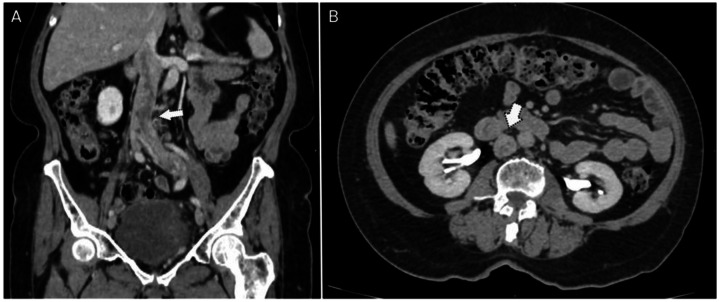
Contrast-enhanced abdominal CT Venous phase (A, B) and delayed phase (C). Filling defect in the left common iliac vein with extension into the inferior vena cava. The left common iliac vein is enlarged compared with the contralateral side, consistent with consistent with thrombosis or intravascular tumor extension (dotted arrow). A: Coronal view. B: Sagittal view. C: Axial view at the infrarenal portio.
